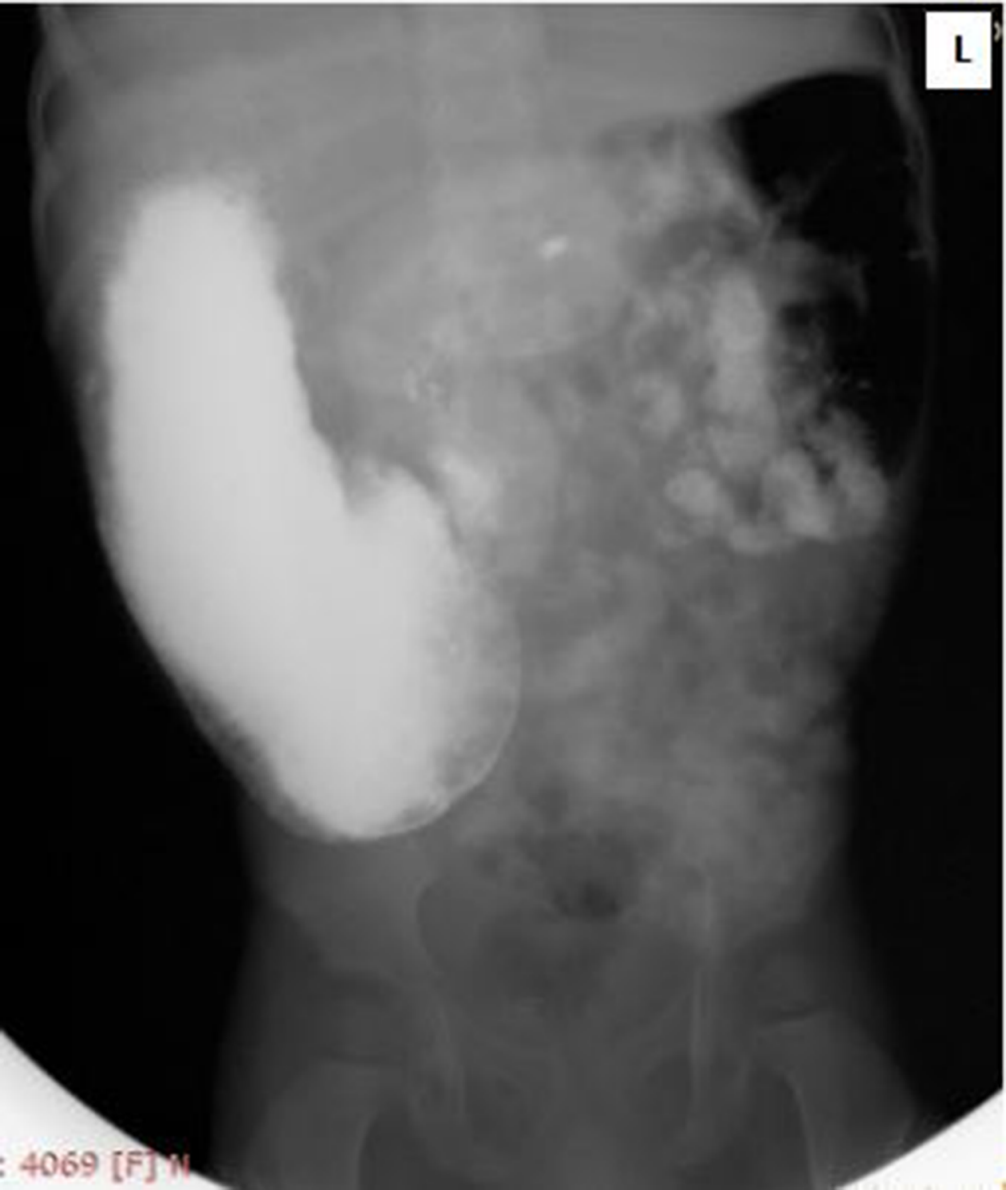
A 2-h barium meal radiograph showing distended stomach with partial pyloric stenosis.
